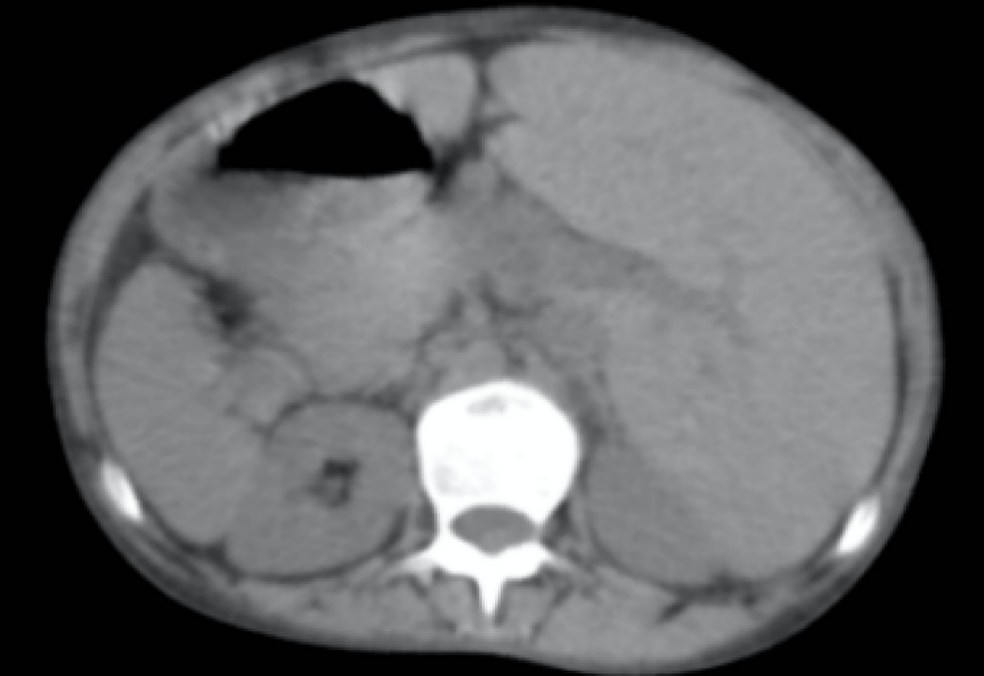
CT of the abdomen showing situs inversus
